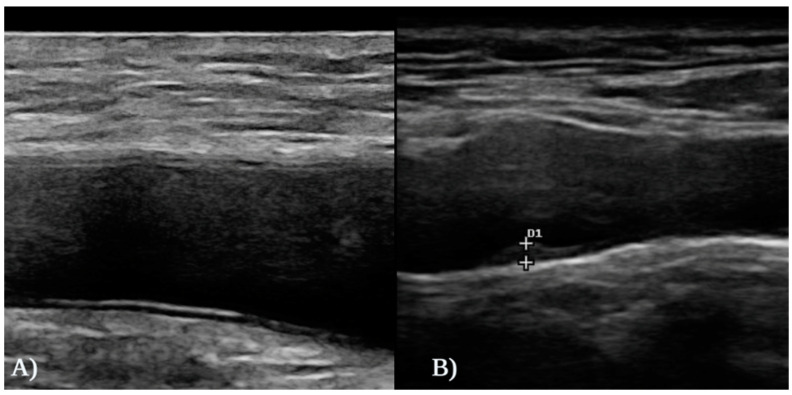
(A) Ultrasound imaging of the right common femoral artery of a healthy control without subclinical atherosclerosis. (B) Atherosclerotic plaque of 1.8 mm detected by ultrasound of the left carotid artery in a patient with AA.
